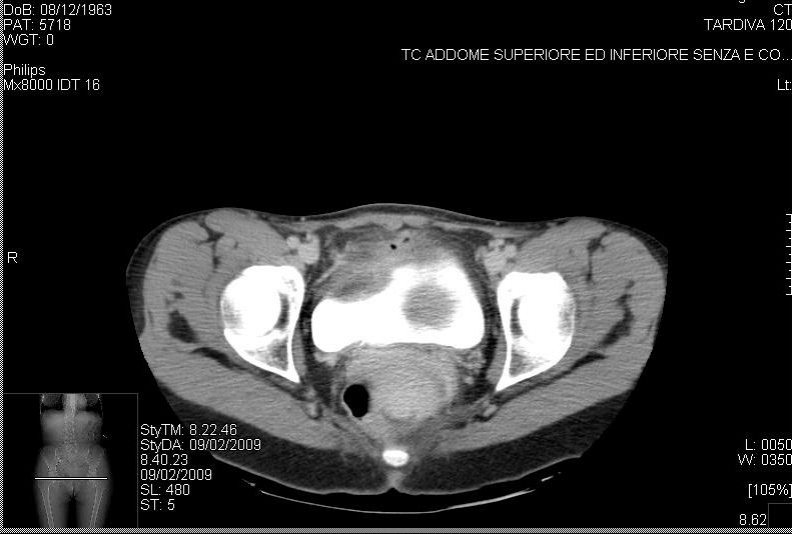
Enhanced abdomen CT scan demonstrate a 6 cm × 2,5 cm anterior bladder mass.
